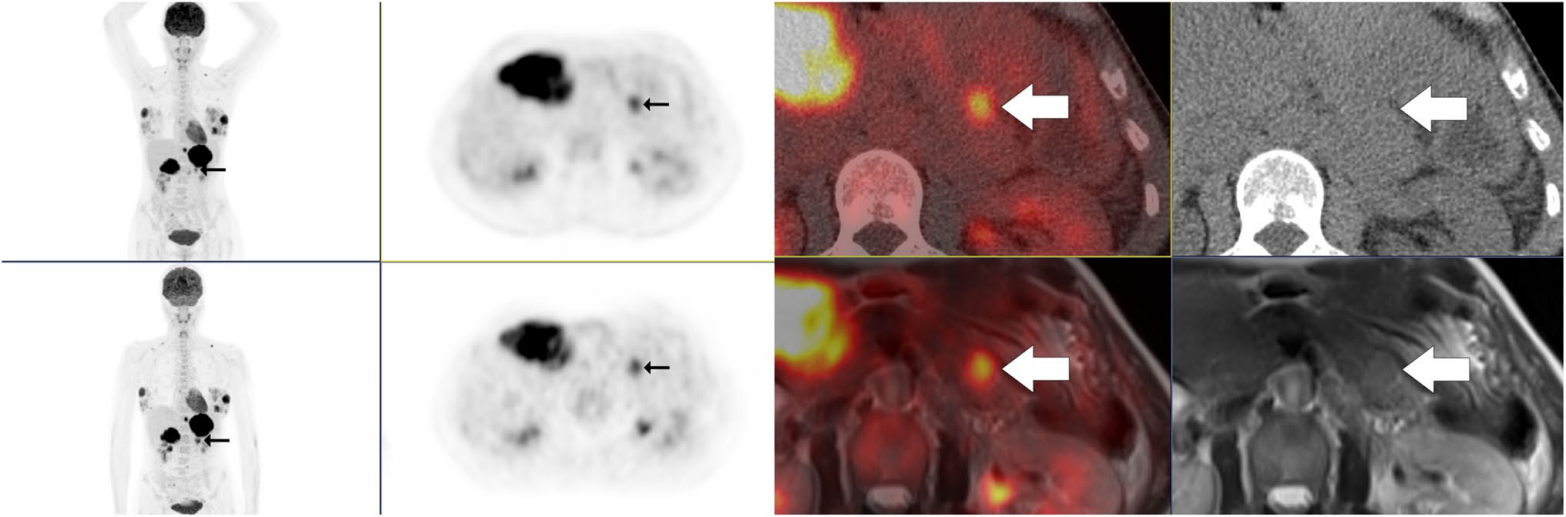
A 48-year-old female with DLBCL stage IVA. Baseline PET/CT in the upper row and PET/MR in the bottom row. On PET/MR T2-HASTE, we see a 18 × 14 mm solid lesion in cauda pancreatic that was scored as a paraaortic lymph node on PET/CT
